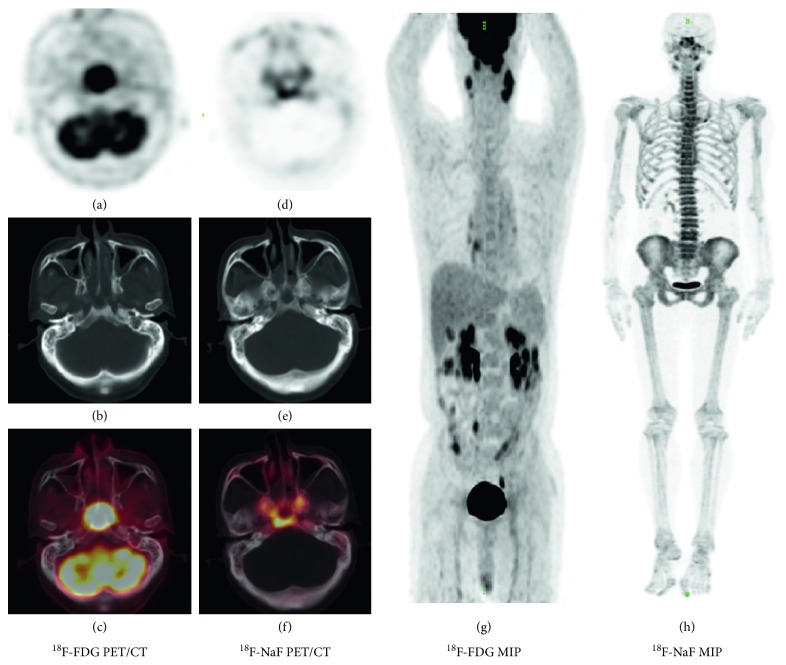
A 63-year-old man was diagnosed with nonkeratinizing nasopharyngeal carcinoma. (A–C) transverse sections of PET, CT, and fusion views in ^18^F-FDG PET/CT, respectively. (D–F) transverse sections of PET, CT, and fusion views in ^18^F-NaF PET/CT. (G and H) the maximum intensity projection (MIP) of ^18^F-FDG PET/CT and ^18^F-NaF PET/CT, respectively. Skull-base invasion was revealed on ^18^F-NaF PET/CT but was hidden on ^18^F-FDG PET/CT because of the interference from the tumor tissue. This was consistent with MRI two days before ^18^F-NaF PET/CT.
